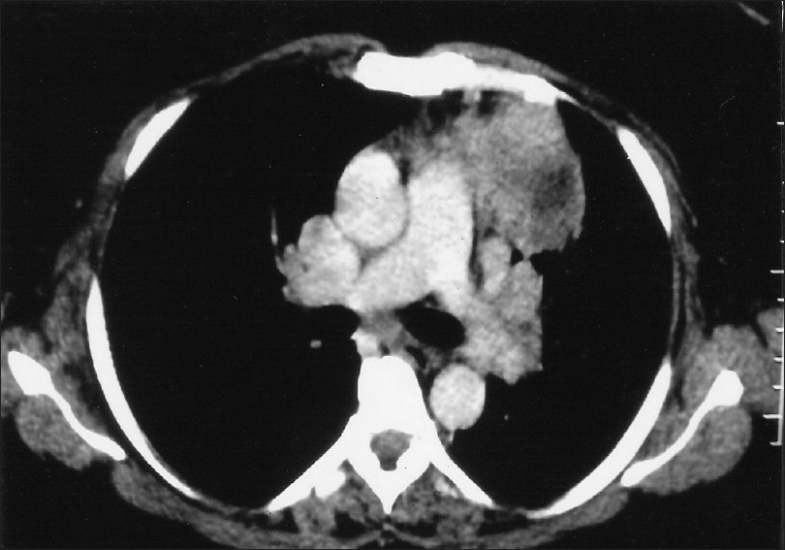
Computed tomography scan of the chest showing heterogeneously enhancing soft tissue density in the left upper lobe
